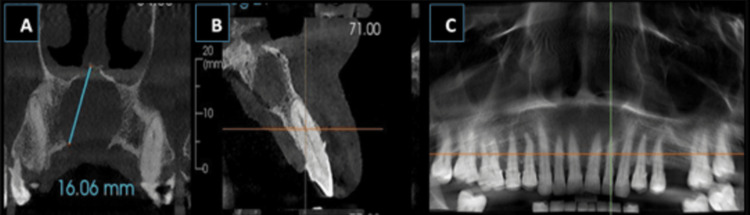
CBCT images showing: A) cystic extension at the maxillary midline; B) palatal relation of the cyst with the tooth root; C) cystic extension to the nasal floor and its involvement CBCT - cone beam computed tomography
